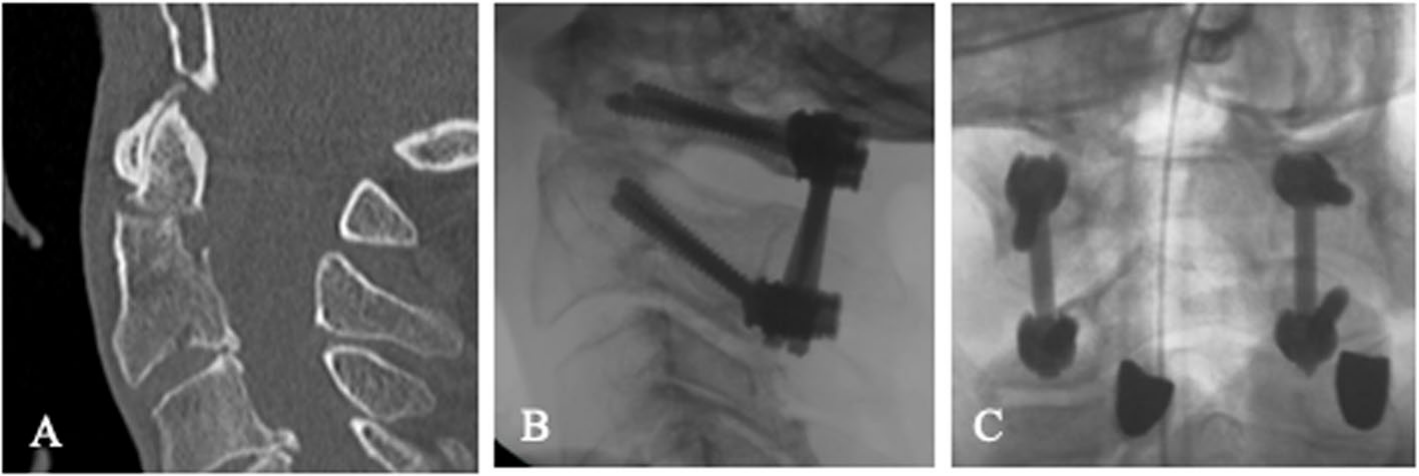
An 95–year–old male patient with odontoid fracture type A according to Eysel and Roosen: Based on the dislocation and given instability, dorsal surgery was performed: Preoperative CT image (A), intraoperative X–ray image (B, C)
